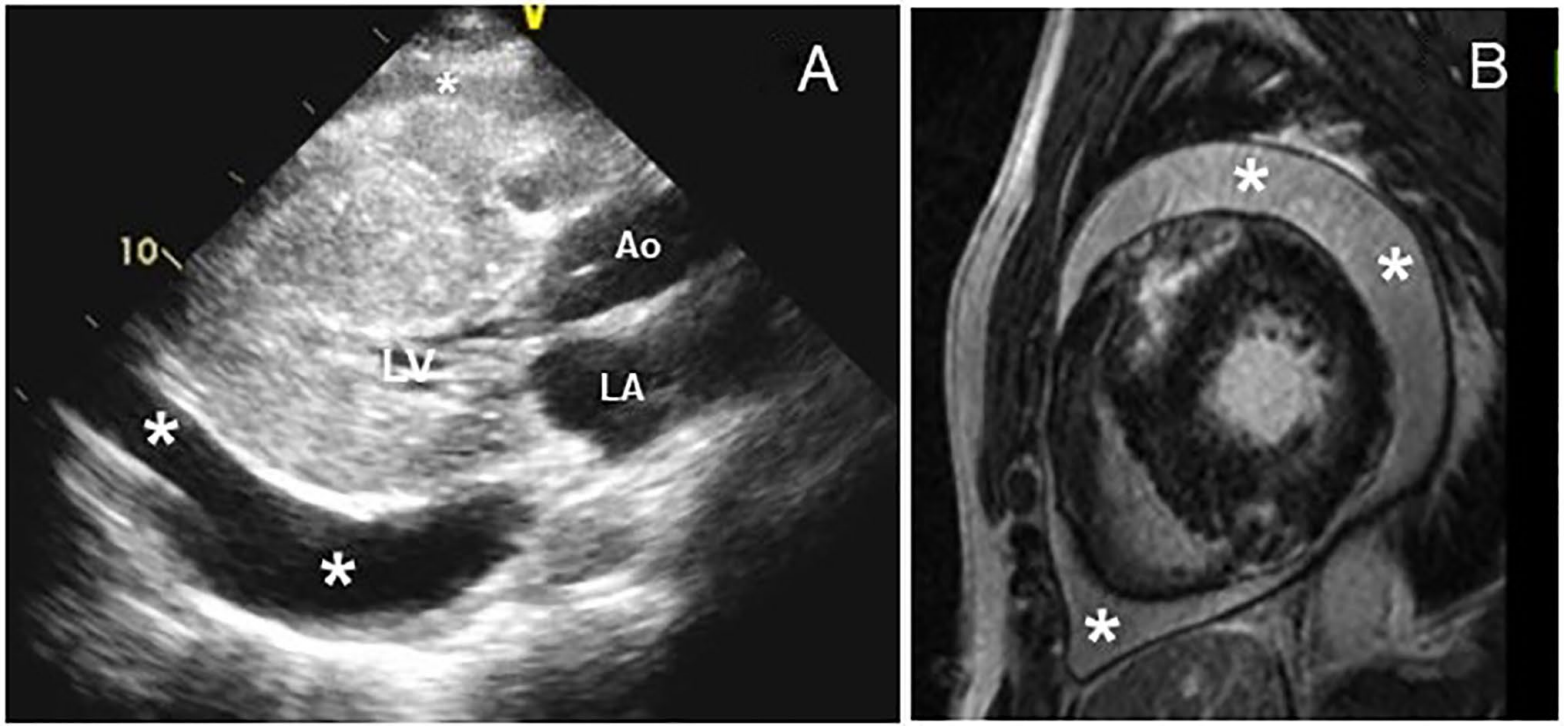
A massive circumferential pericardial effusion (asterisks) in a 40-year-old man with a hypertrophic cardiomyopathy (patient #2) demonstrated by transthoracic echocardiogram (a) and cardiovascular magnetic resonance imaging (b)
